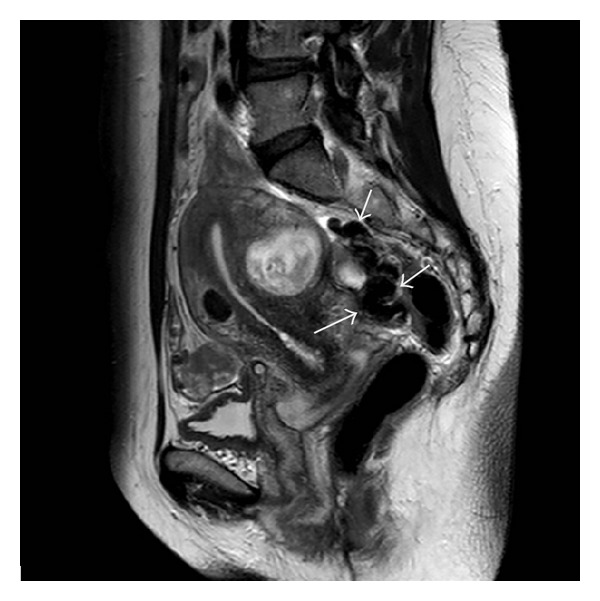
Sagittal T2-weighted MR image shows polylobular masses in the pouch of Douglas with very low signal intensity.
